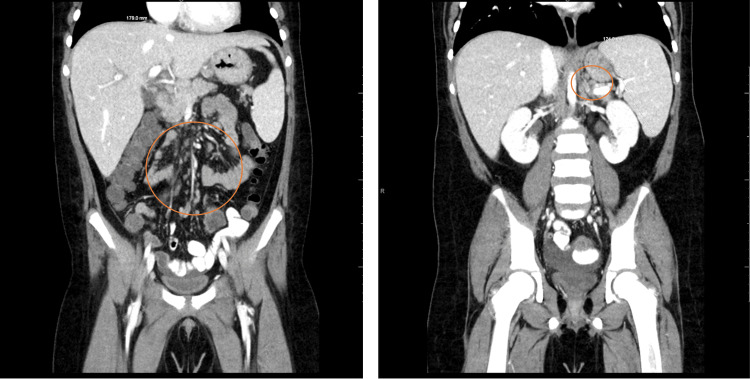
Coronal CT of the abdomen and pelvis demonstrating liver and spleen dimensions (hepatosplenomegaly) and numerous enlarged lymph nodes
